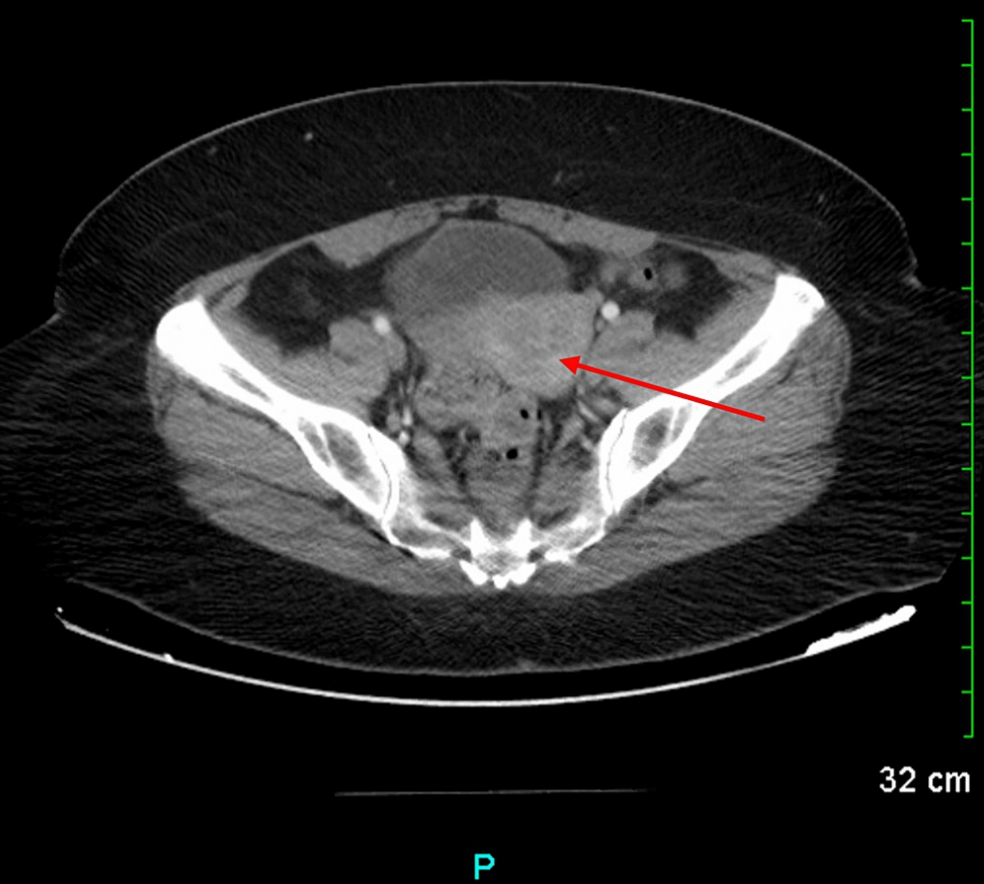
CT scan of the abdomen and pelvis with contrast taken prior to the patient’s endometrial ablation procedure The red arrow shows a lobular contour to the uterus suggestive of leiomyomas.
